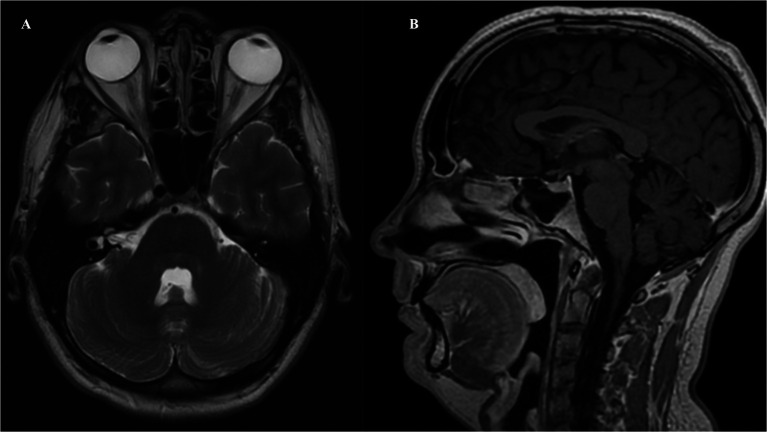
Brain MRI of patient 3. (A) Axial T2-weighted image showing mild enlargement of the fourth ventricle. (B) Midline sagittal T1-weighted image demonstrating mild atrophy of the rostral cerebellar vermis.
